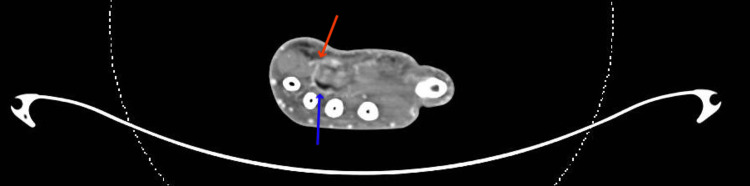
CT angiography The patency of the superficial palmar arch and the deep palmar arch are displayed. The red arrow indicates the superficial arch, while the blue arrow indicates the deep arch.
